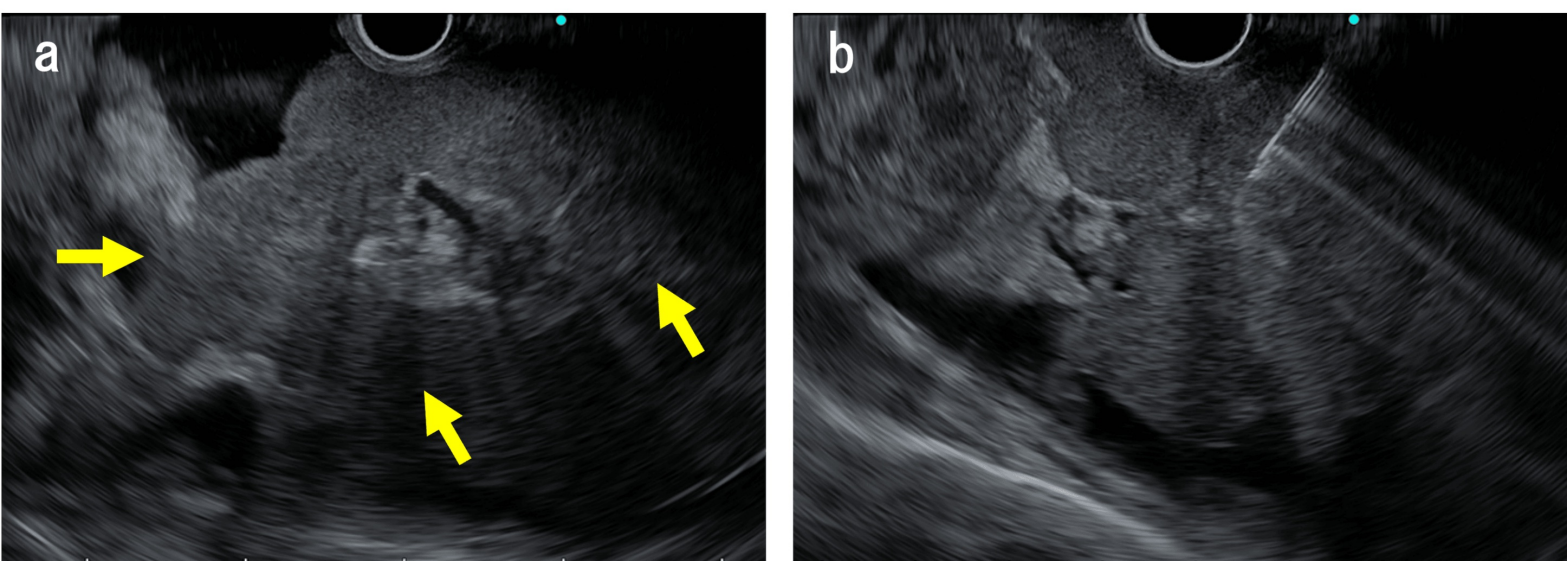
Endoscopic ultrasonography images (a) The exophytic gastric tumor showing a uniformly hypoechoic mass (arrows). (b) Endoscopic ultrasound-guided fine-needle biopsy is performed for definitive diagnosis.
